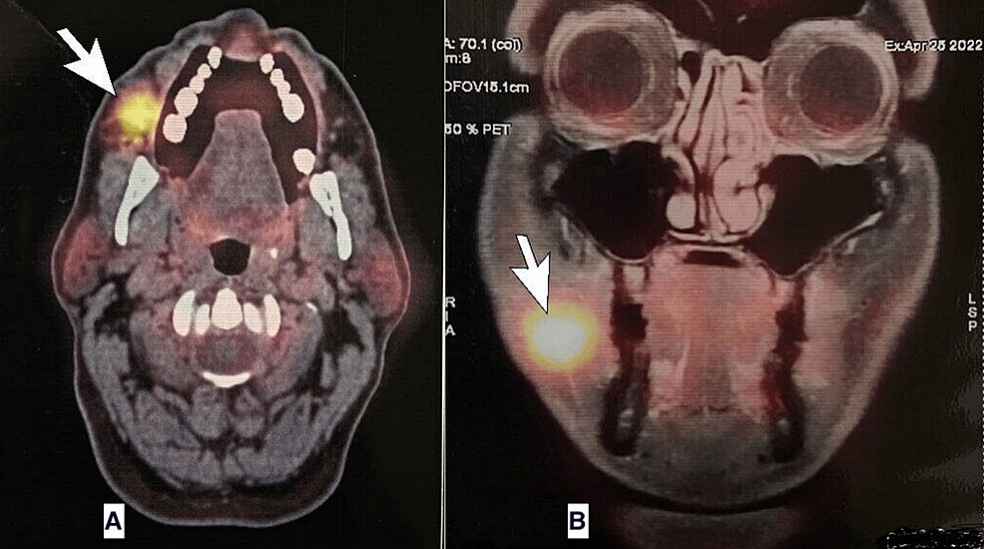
Positron emission tomography-CT with fusion MRI scan of the head showing fluorodeoxyglucose-avid lesions in right buccal mucosa marked by white arrows
